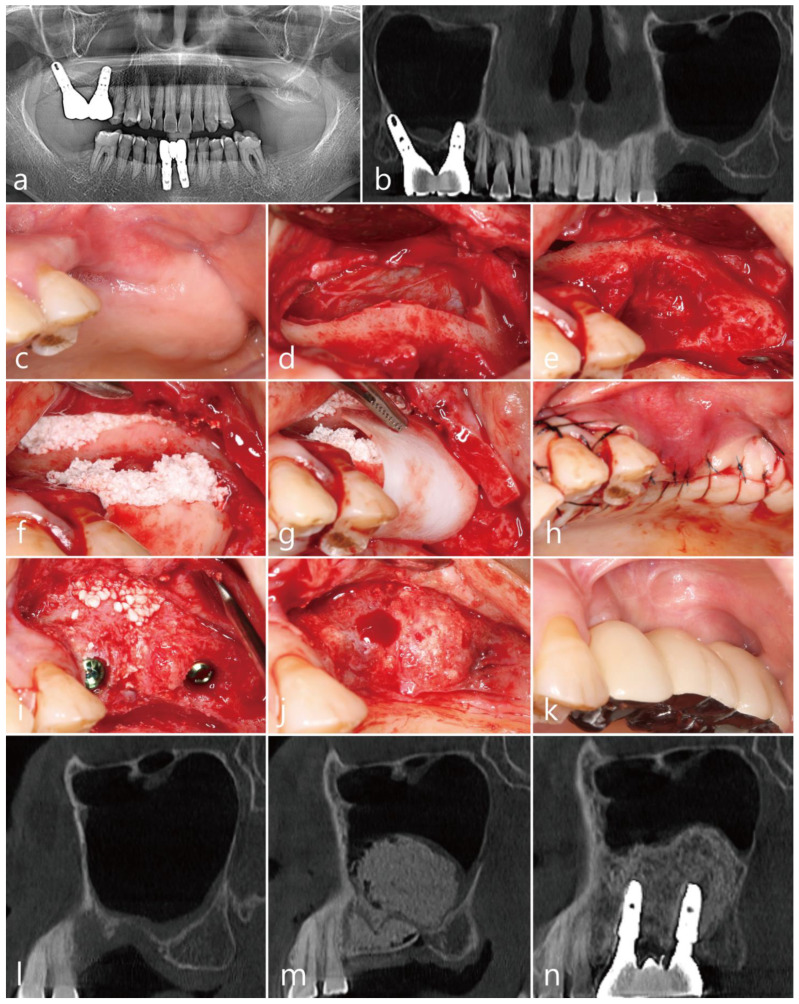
Case 2: (a) preoperative panoramic radiograph; (b) preoperative panoramic image of the CBCT scan showing a severely resorbed bone defect and a pneumatized maxillary sinus on the left side; (c) preoperative clinical view at two months after extraction of the left maxillary second premolar and first molar; (d) after reflection of the mucoperiosteal flap, the lateral bony window was obtained; (e) thorough debridement was performed to remove inflammatory granulation tissue; (f) after maxillary sinus augmentation, an appropriately trimmed lateral bony window was placed on the defect, and the gap was filled with particulate bone graft; (g) the grafted site was covered with a collagen membrane; (h) the mucoperiosteal flaps were closed with minimal tension; (i) implants were placed six months after the surgery, and additional bone grafting was performed at the lateral window site where bone loss had occurred due to the sinus graft infection; (j) the uncovering procedure was performed six months after implant placement; (k) a prosthesis was delivered two months after the uncovering procedure; (l) preoperative sagittal image of the CBCT scan; (m) sagittal images of the CBCT taken immediately after surgery; (n) sagittal image of the CBCT one year after prosthesis delivery.
